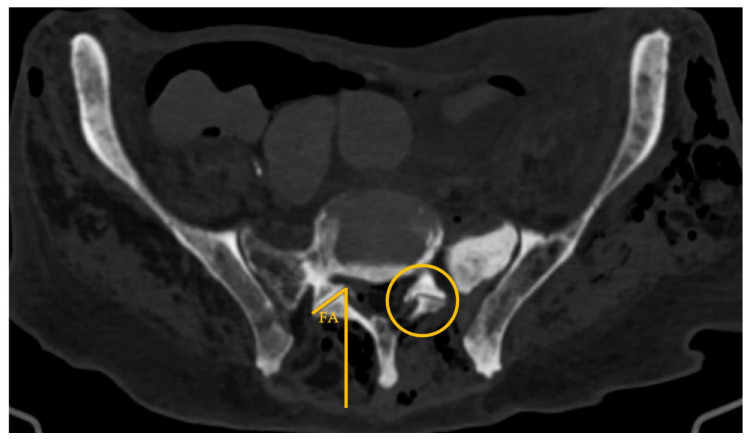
Lumbosacral spine CT—axial plane; circle: highlights the location of the left L5–S1 facet (zygapophyseal) joint; FA: facet joint angulation, as defined by the yellow solid lines.
